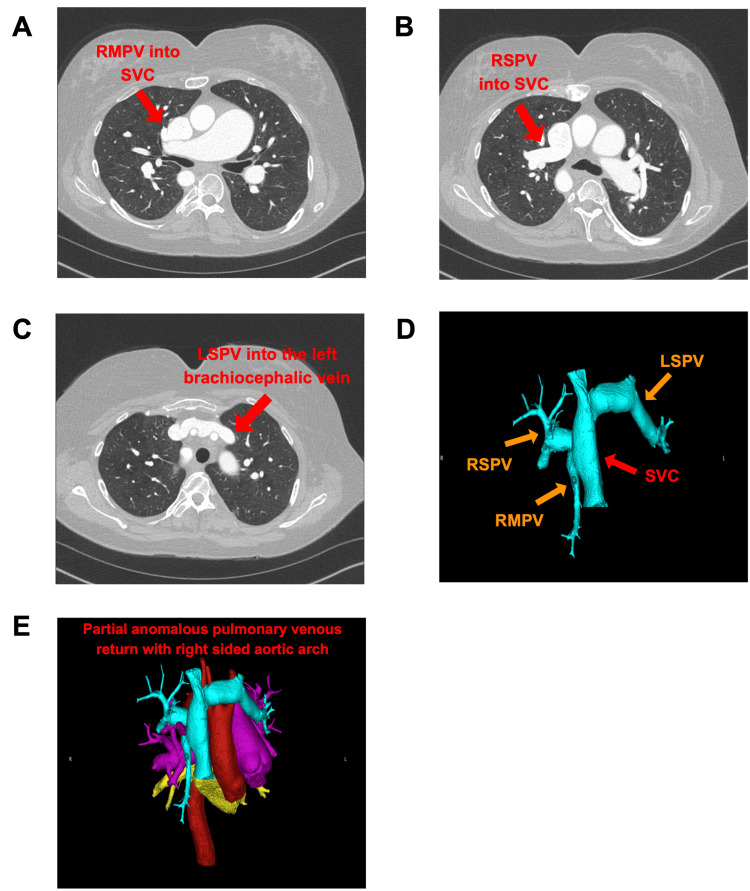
Chest CT and 3D reconstruction confirming PAPVR in TS. (A) Right middle pulmonary vein draining into the SVC. (B) Right superior pulmonary vein draining into the SVC. (C) Left superior pulmonary vein draining into the left brachiocephalic vein. (D) 3D reconstructed image demonstrating the connection of the pulmonary veins with the SVC (cyan). (E) 3D reconstructed image showing PAPVR with the SVC (cyan), aortic arch (red), and pulmonary veins (magenta). LSPV, left superior pulmonary vein; PAPVR, partial anomalous pulmonary venous return; RMPV, right middle pulmonary vein; RSPV, right superior pulmonary vein; SVC, superior vena cava; TS, Turner syndrome
